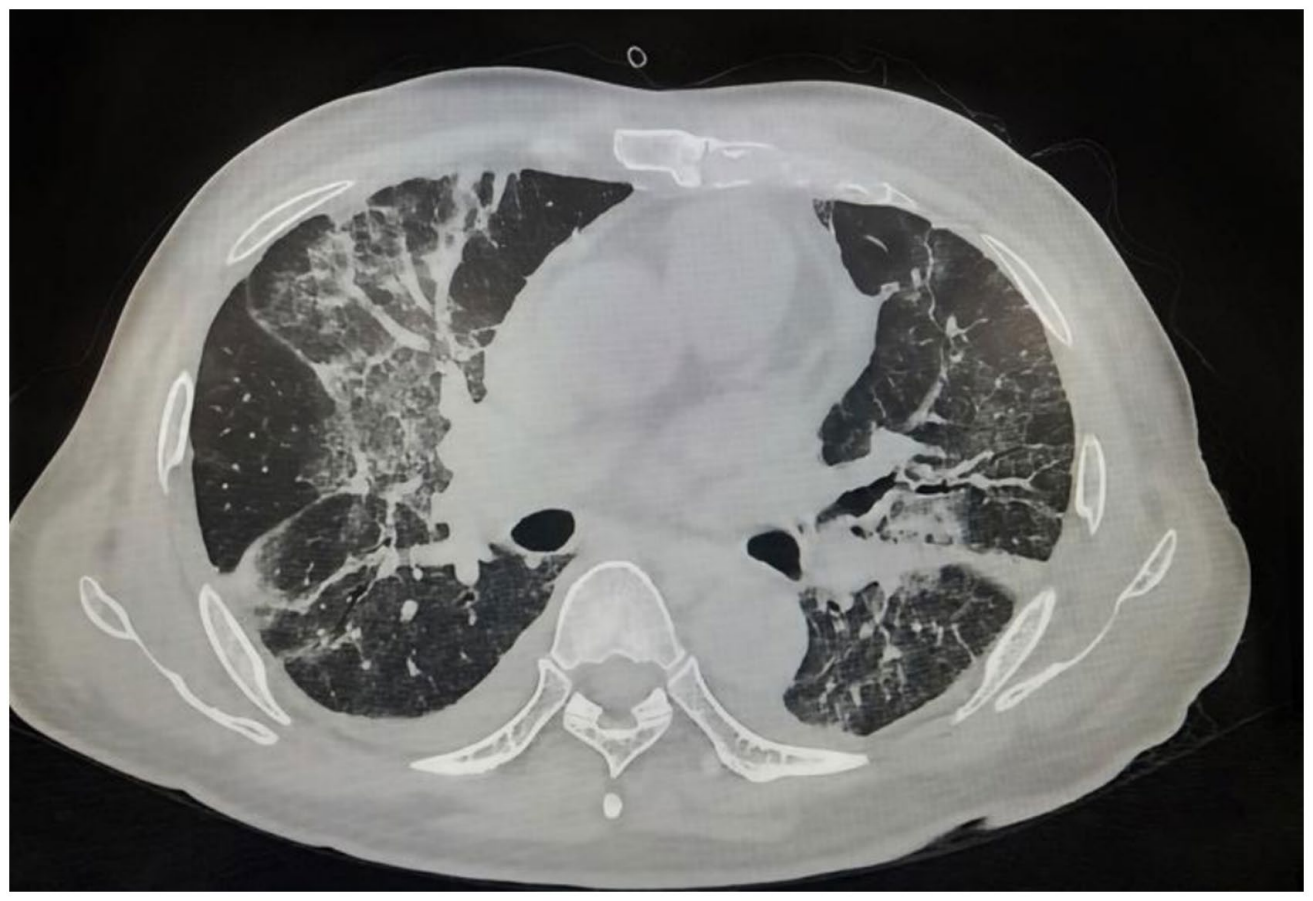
Chest CT demonstrated multifocal ground-glass opacities, linear opacities, and patchy consolidation bilaterally, suggesting an infectious etiology.
